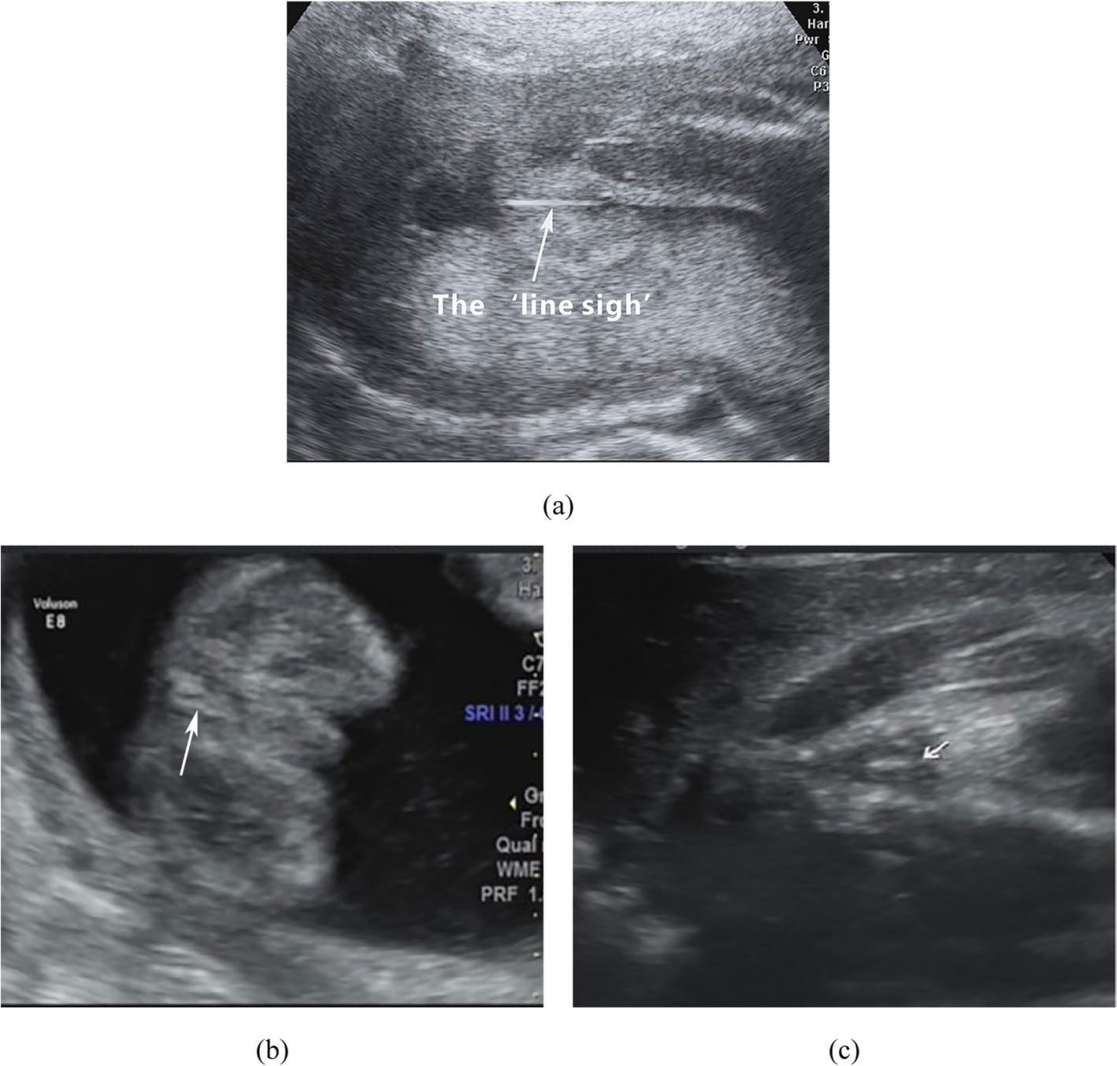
The sonographic demonstration of the anus of the fetus with imperforate anus showed ‘line sign’. The higher the position of the imperforate anus was, the more obvious the ‘line sign’. a High type imperforate anus. b Imperforate anus between high and low position. c Low type imperforate anus
